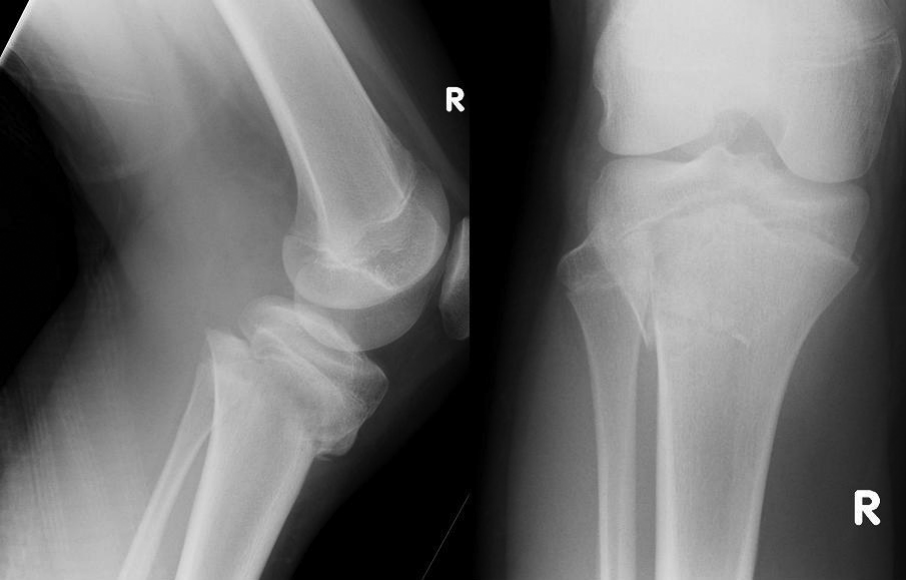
Pre-operative radiographs.
